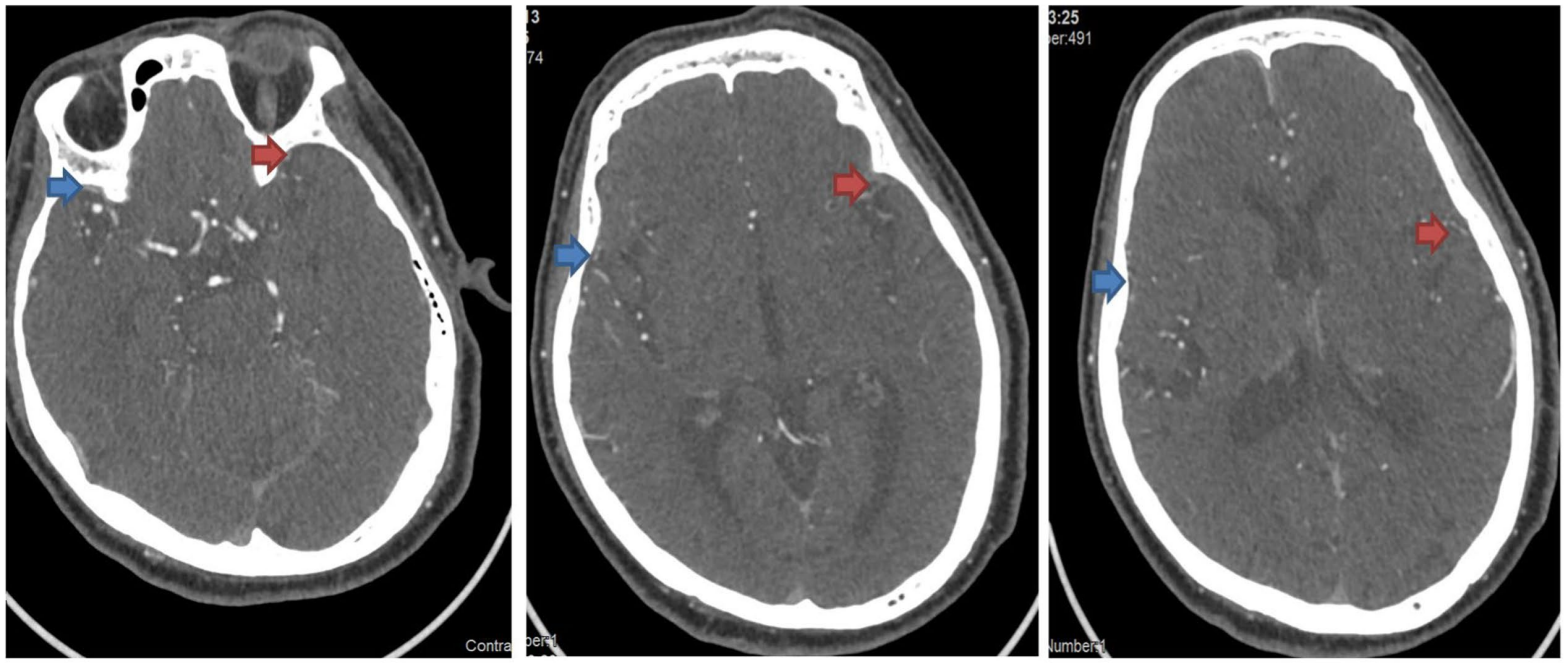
Assessment of venous outflow on the CTA images of Case 2. The side indicated by the red arrow is the affected side, and the side indicated by the blue arrow is the healthy side. From left to right in the figure, the COVES scores are as follows: SPS is 2 point, SMA is 1 point, LA is 2 point, and the total score is 5 point.
